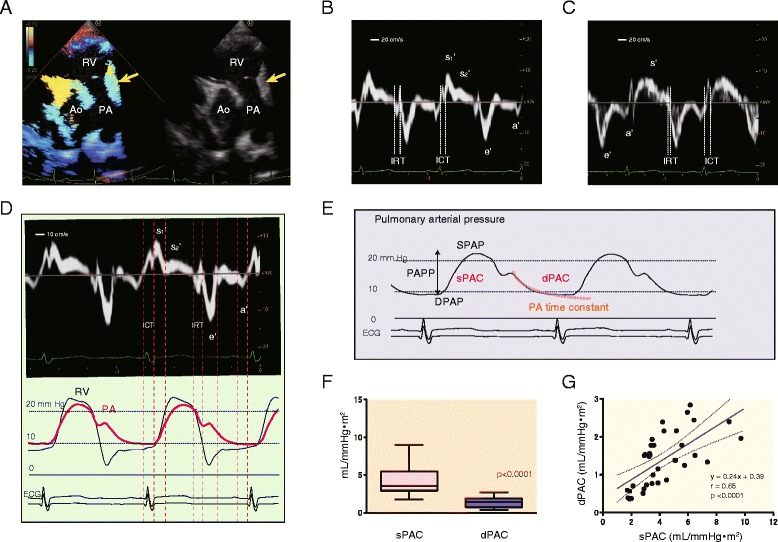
Recording of pulmonary annular motion velocity and measurement of pulmonary arterial capacitance. A representative recording of pulmonary annular motion evaluated by tissue Doppler imaging in a healthy 2-year-old boy is shown. The long-axis view of the right ventricular outflow tract and main pulmonary artery (PA) is visualized, and the sample volume is positioned on the RV free wall side of the pulmonary annulus, as indicated by the *yellow arrow* (a). Pulmonary annular velocity of the RV free wall side is determined (b). The tricuspid annular motion waveform from the same individual is also evaluated for the sake of comparison (c). The tissue Doppler-derived annular velocity waveform comprises s1′, s2′, e’, and a’ for the pulmonary annulus, and s’, e’, and a’ for the tricuspid annulus. Simultaneous recordings of pulmonary annular motion and RV and PA pressure curves in a 4-year-old girl with a ventricular septal defect (VSD) are shown (d). The measurements of systolic pulmonary arterial capacitance (sPAC) and diastolic pulmonary arterial capacitance (dPAC) are shown (e). The calculations to obtain sPAC and dPAC are described in the methods section. The comparison between sPAC and dPAC is shown in panel f. *Boxes* show the distribution (25th and 75th percentiles; central line, median). *Vertical lines* represent the range between the 5th and 95th percentiles. The relationship between sPAC and dPAC is shown in panel g. Ao, aorta; PA, pulmonary artery; RV, right ventricle; ICT, isovolumic contraction time; IRT, isovolumic relaxation time; sPAC, systolic pulmonary arterial capacitance; dPAC, diastolic pulmonary arterial capacitance; SPAP, systolic pulmonary arterial pressure; DPAP, diastolic pulmonary arterial pressure; PAPP, pulmonary arterial pulse pressure
